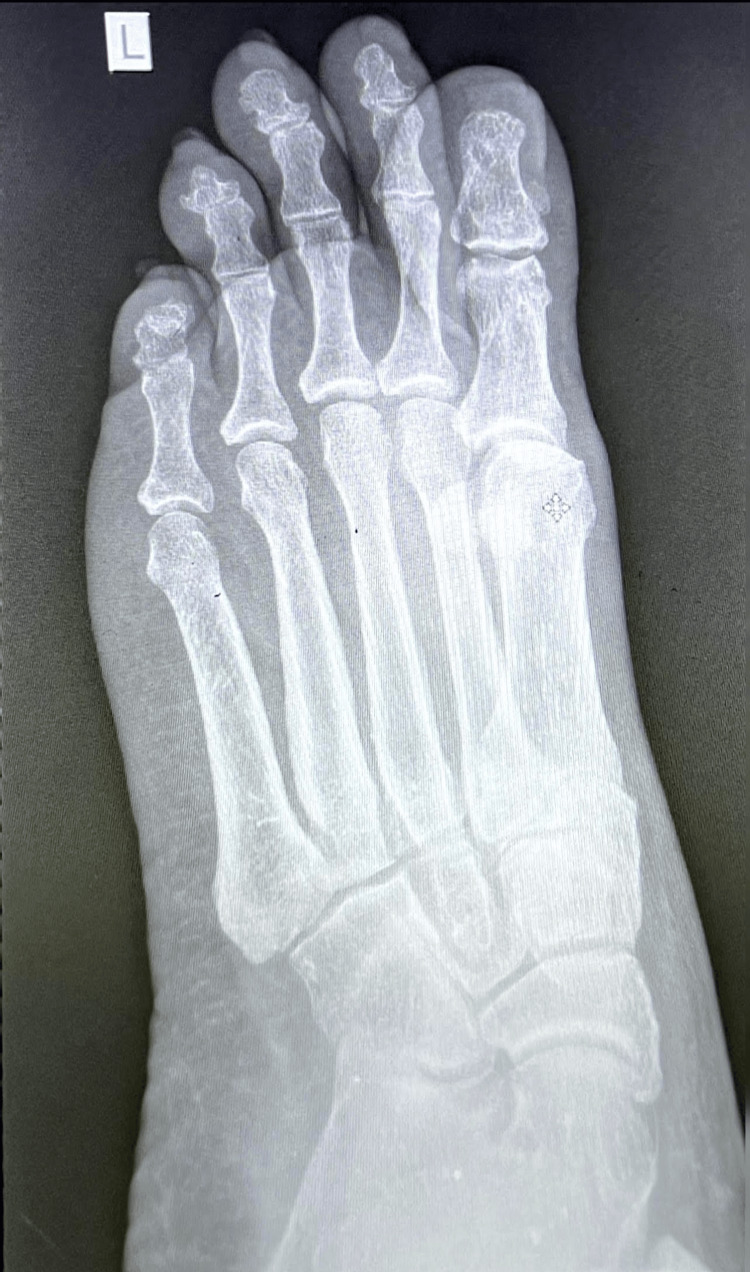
Initial AP plain X-ray of the left foot demonstrating no evidence of fracture. AP: anteroposterior.
